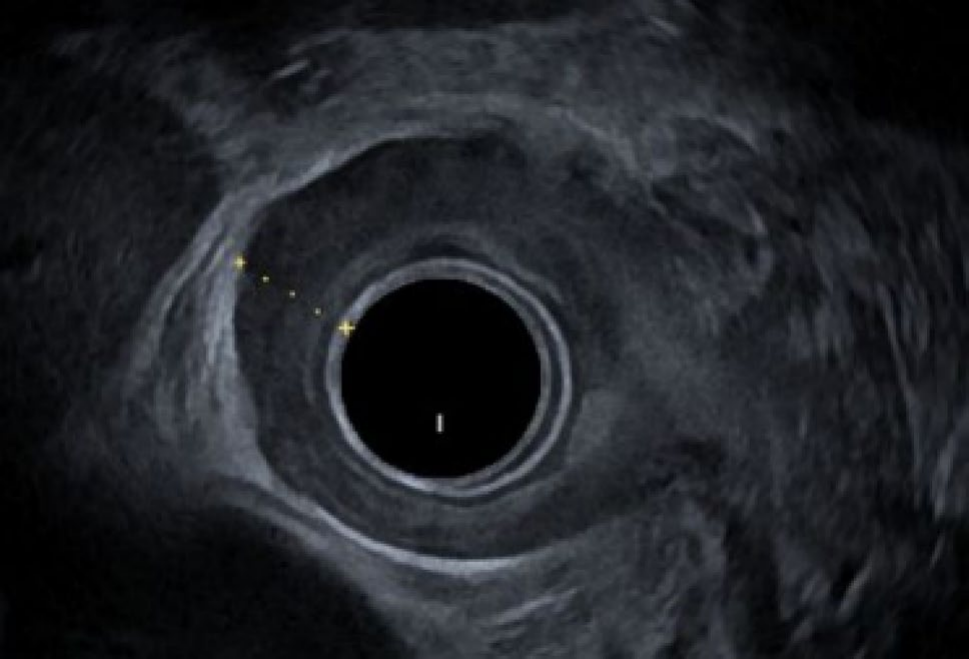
Upper endoscopic ultrasound (EUS) with visualization of the esophageal wall in the lower esophagus
